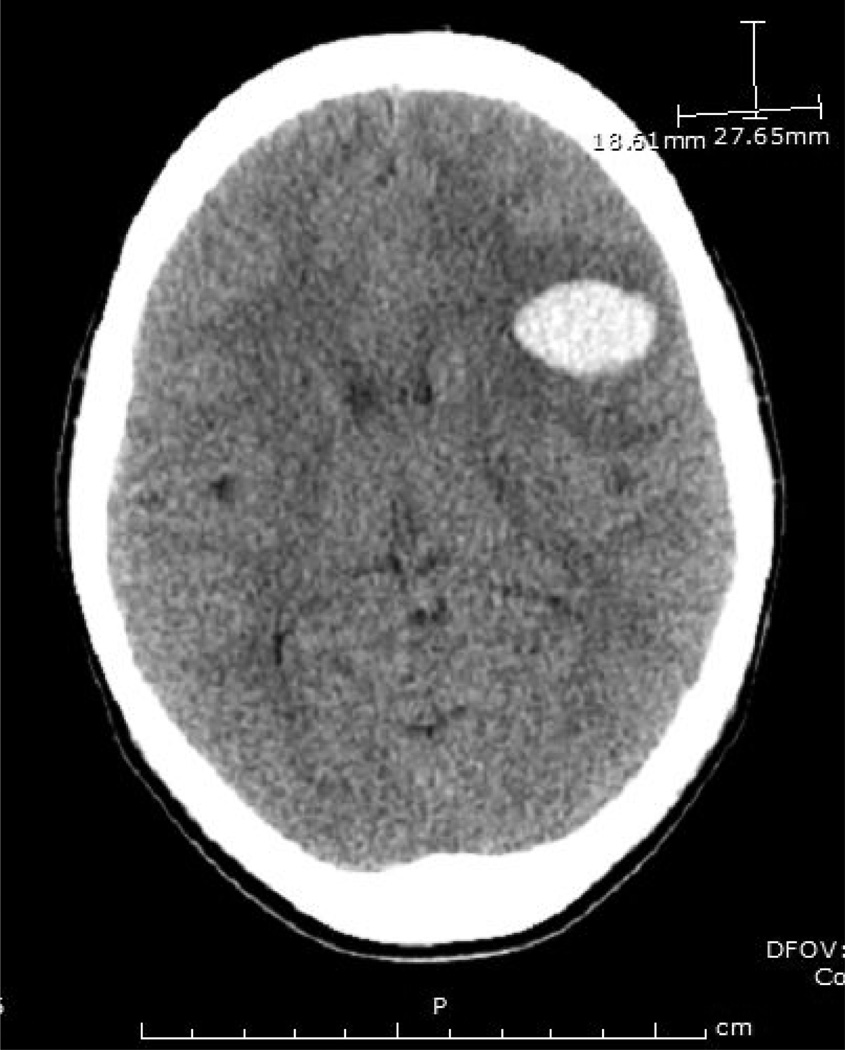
Emergent head CT demonstrating the larger of two frontoparietal hemorrhages.
